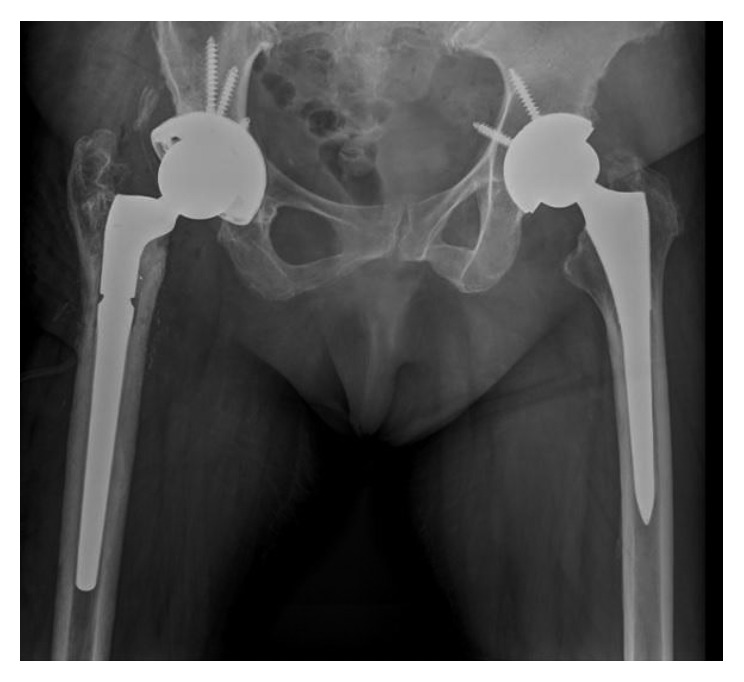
Radiograph after one-stage replacement for acute periprosthetic infection on July 2014.
